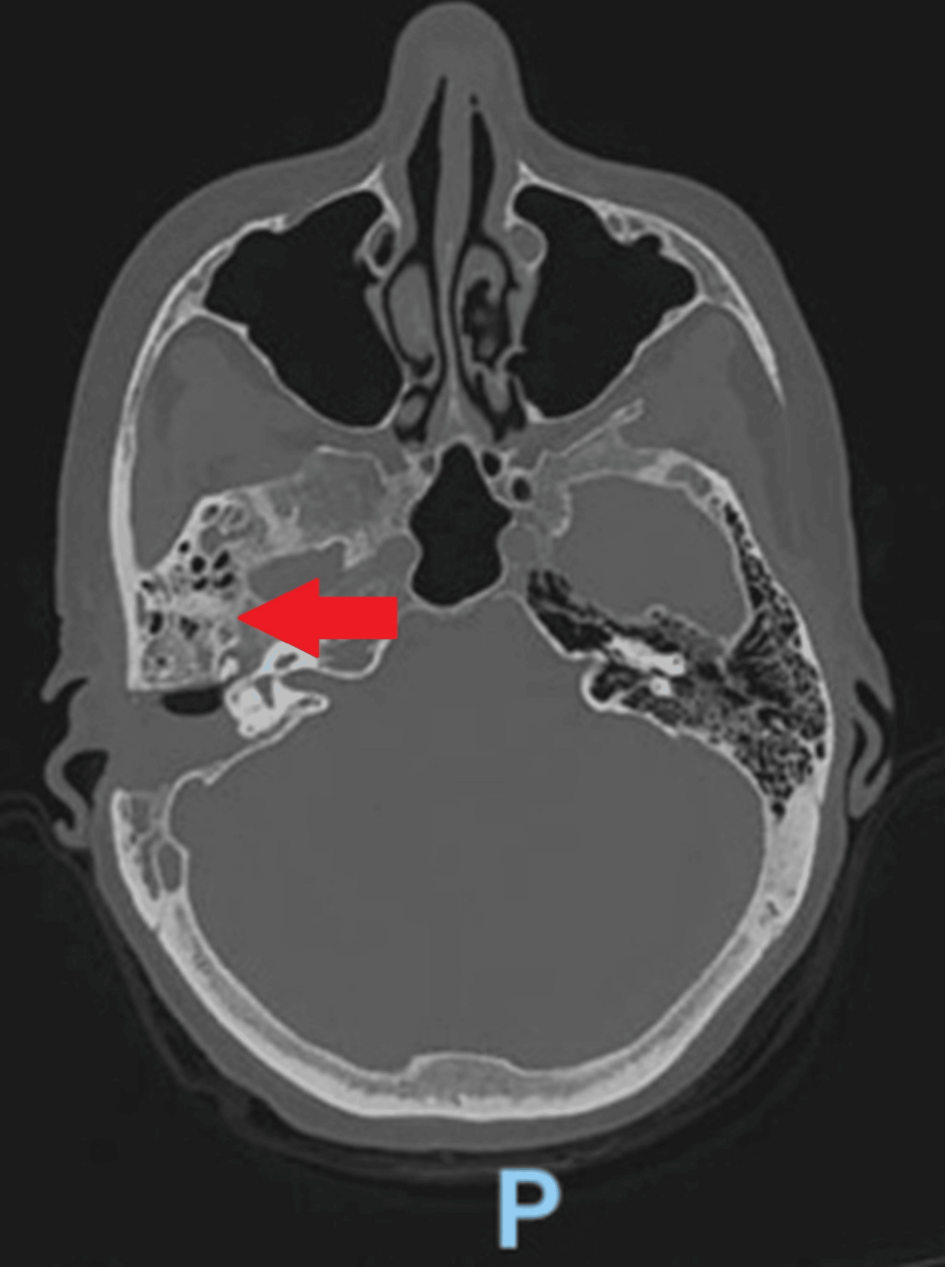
CT scan of the head and ear. The study shows an image of right-sided otomastoiditis with associated morphological changes in the posterior mastoid air cells and the mastoid tip (red arrow).
